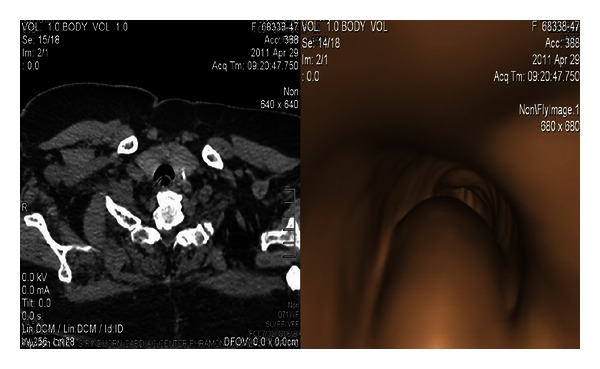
Chest dynamic multislice CT scan and CT virtual bronchoscopy showing collapse of trachea at posterior wall.
